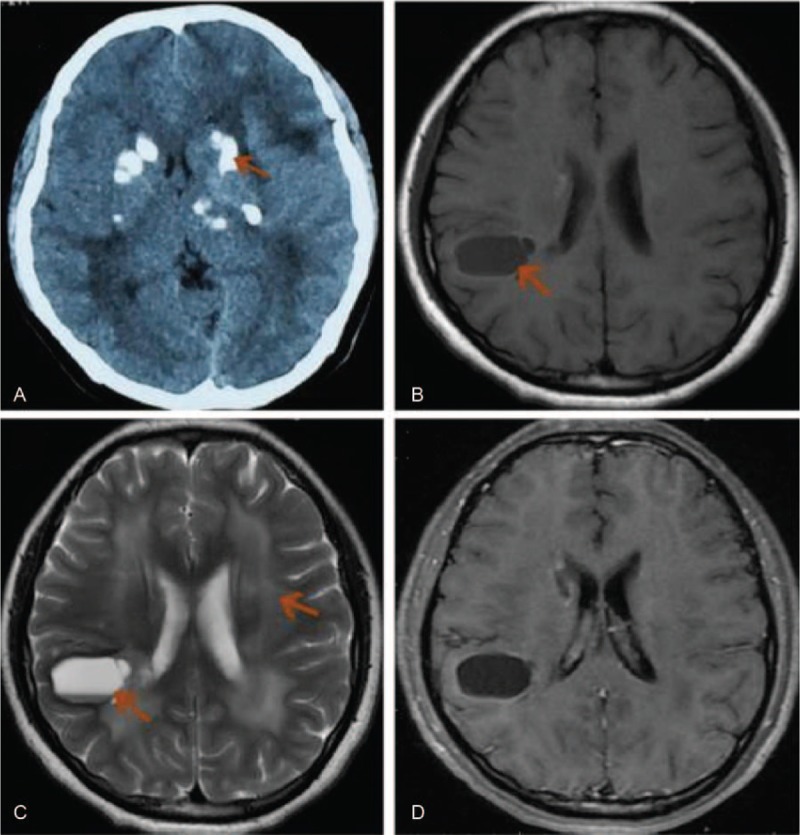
Radiological profiles of Case 2. (A) Brain computed tomography showed multiple asymmetric calcifications in the bilateral basal ganglia and white matter. (B) Magnetic resonance T1-weighted imaging showed a cyst in the right parietal lobe with deposit of hemosiderin. (C) Magnetic resonance T2-weighted imaging showed a giant cyst in the right parietal lobe and hyperintensities in the bilateral white matter. (D) Magnetic resonance contrasted T1-weighted imaging showed ring-like enhancement along the cyst wall.
